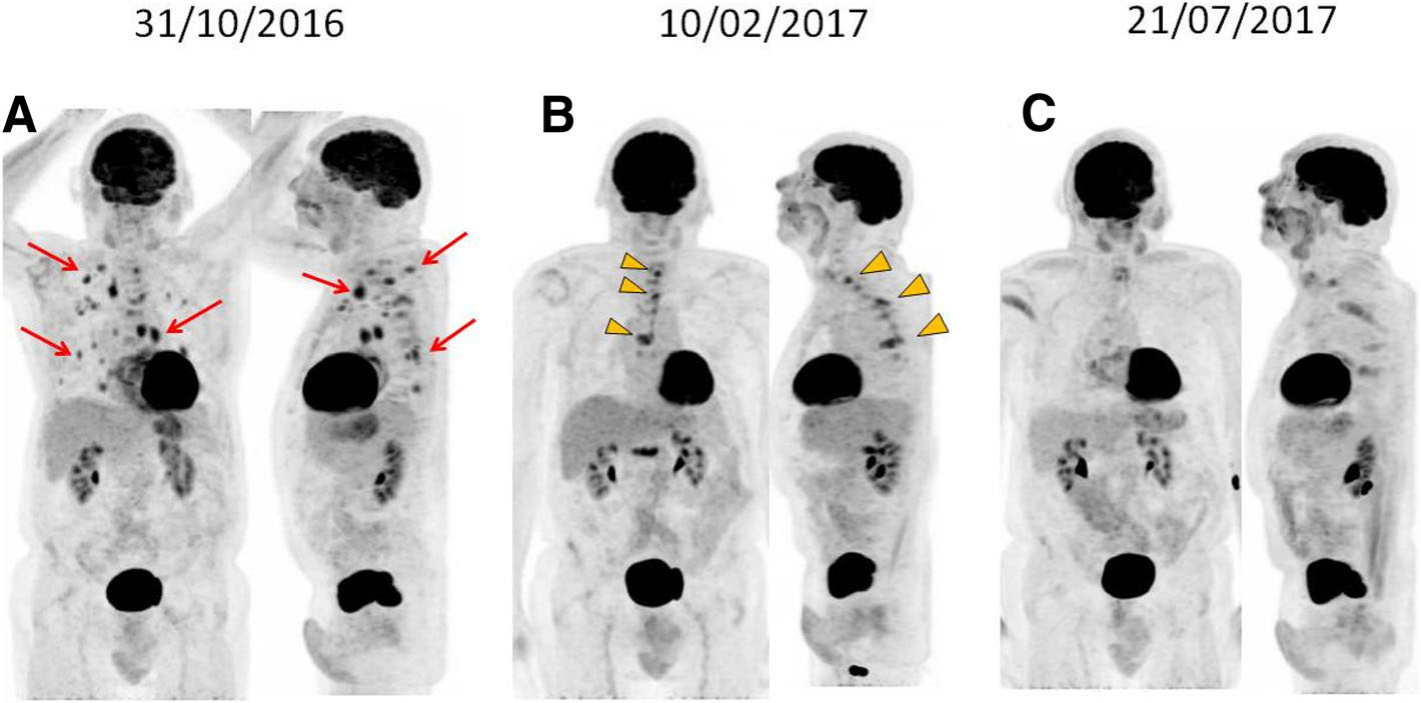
Evolution of pulmonary, mediastinal and vertebral lesions seen in ^18^F-FDG PET/CT’s MIP images during treatment with anti-PD1. MIP images (front and lateral view) from different ^18^F-FDG PET/CT’s. a Multiple FDG avid lesions in the lungs, mediastinum and thoracic vertebral bodies. b Metabolic resolution of the metastatic lung and mediastinal lesions, however there is increased FDG uptake in previous thoracic bone lesions associated with new hypermetabolic bone lesion (arrowhead). c Metabolic resolution of most thoracic bone lesions, consistent with partial metabolic response (PMR)
